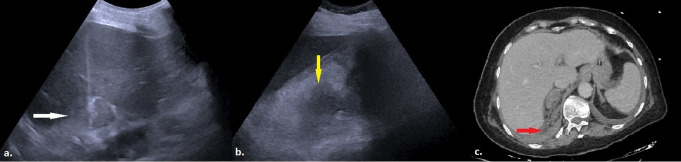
a) The image shows an ultrasound view obtained immediately after a biopsy performed via CTHa of a mass in the right adrenal region (white arrow). b) A control US examination performed during the observation period, revealed a heterogeneous hypoechoic area in the right retroperitoneum (yellow arrow), corresponding to the biopsy site, with an indistinct border from the lesion. c) An abdominal CT scan demonstrated hemorrhage in the retroperitoneal area surrounding the biopsied mass (red arrow). Pathological examination of the lesion confirmed metastasis of lung adenocarcinoma (CTHa: Complete Transhepatic Approach, US: Ultrasound; CT: Computed Tomography)
